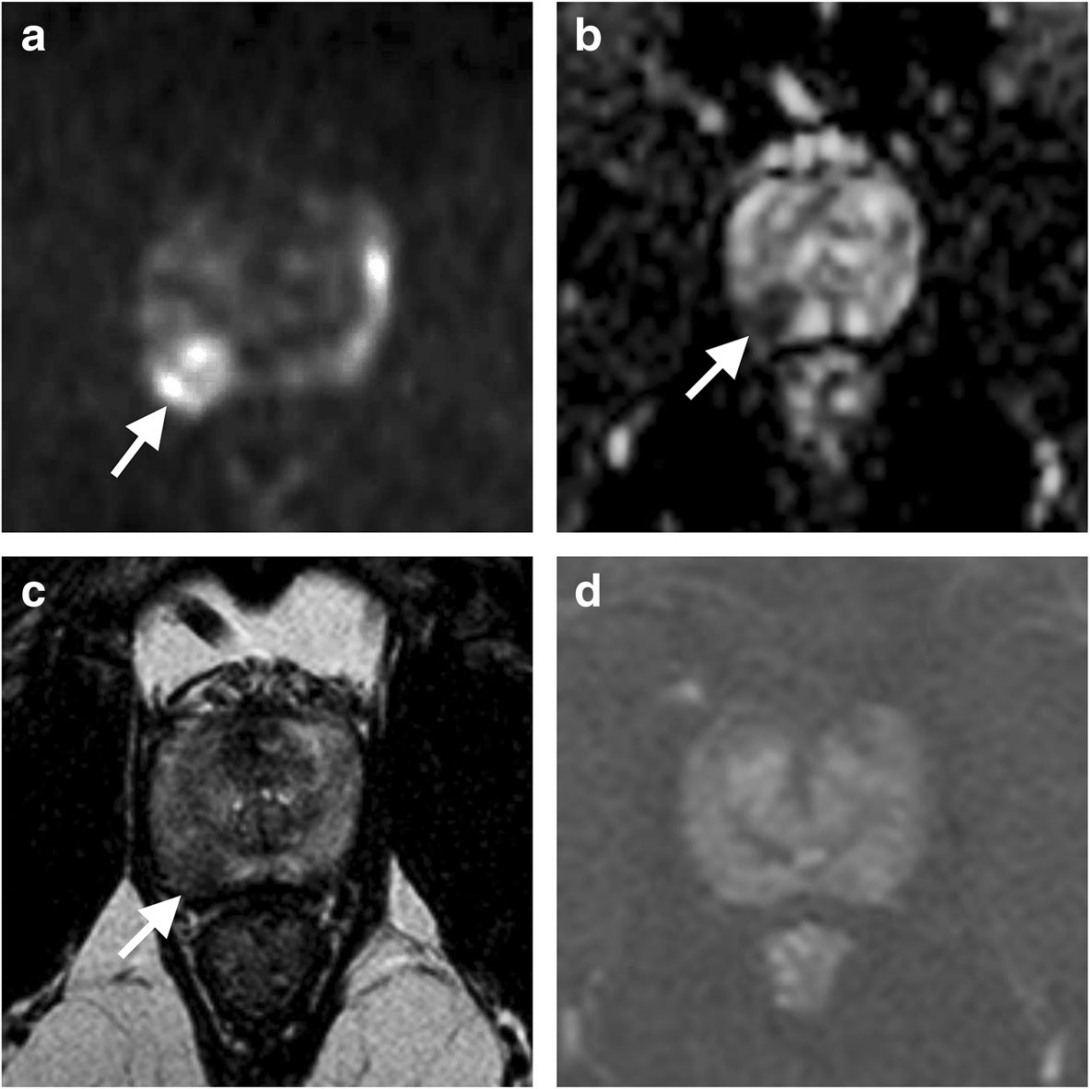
Example case of mpMRI in which the information given by dynamic contrast-enhanced (DCE) imaging was redundant. A 54-year-old man with slightly elevated PSA level (3.43 ng/mL) and suspicious digital rectal examination underwent the examination to target biopsy, showing right midglandular < 15 mm in size PZ finding categorised as PI-RADS 4 because of focal restricted diffusion well visible as hyperintensity on the high *b* value image (a) and hypointensity on the ADC map (b), associated to hypointensity on a transverse T2-weighted image (arrows in c). Cancer was proven by biopsy and then by pathology after surgery with Gleason score 7 (4 + 3). Of note, transverse subtracted DCE (d) had no role in detecting and localising cancer, showing no differences in contrast enhancement compared to the surrounding PZ
